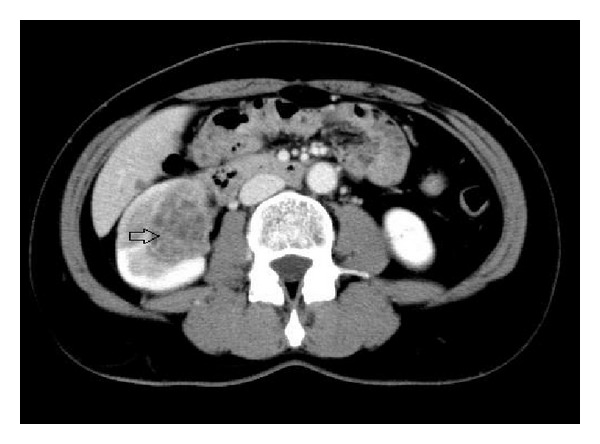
CT scan image of the abdomen showing the tumour (arrow) in the right kidney.
